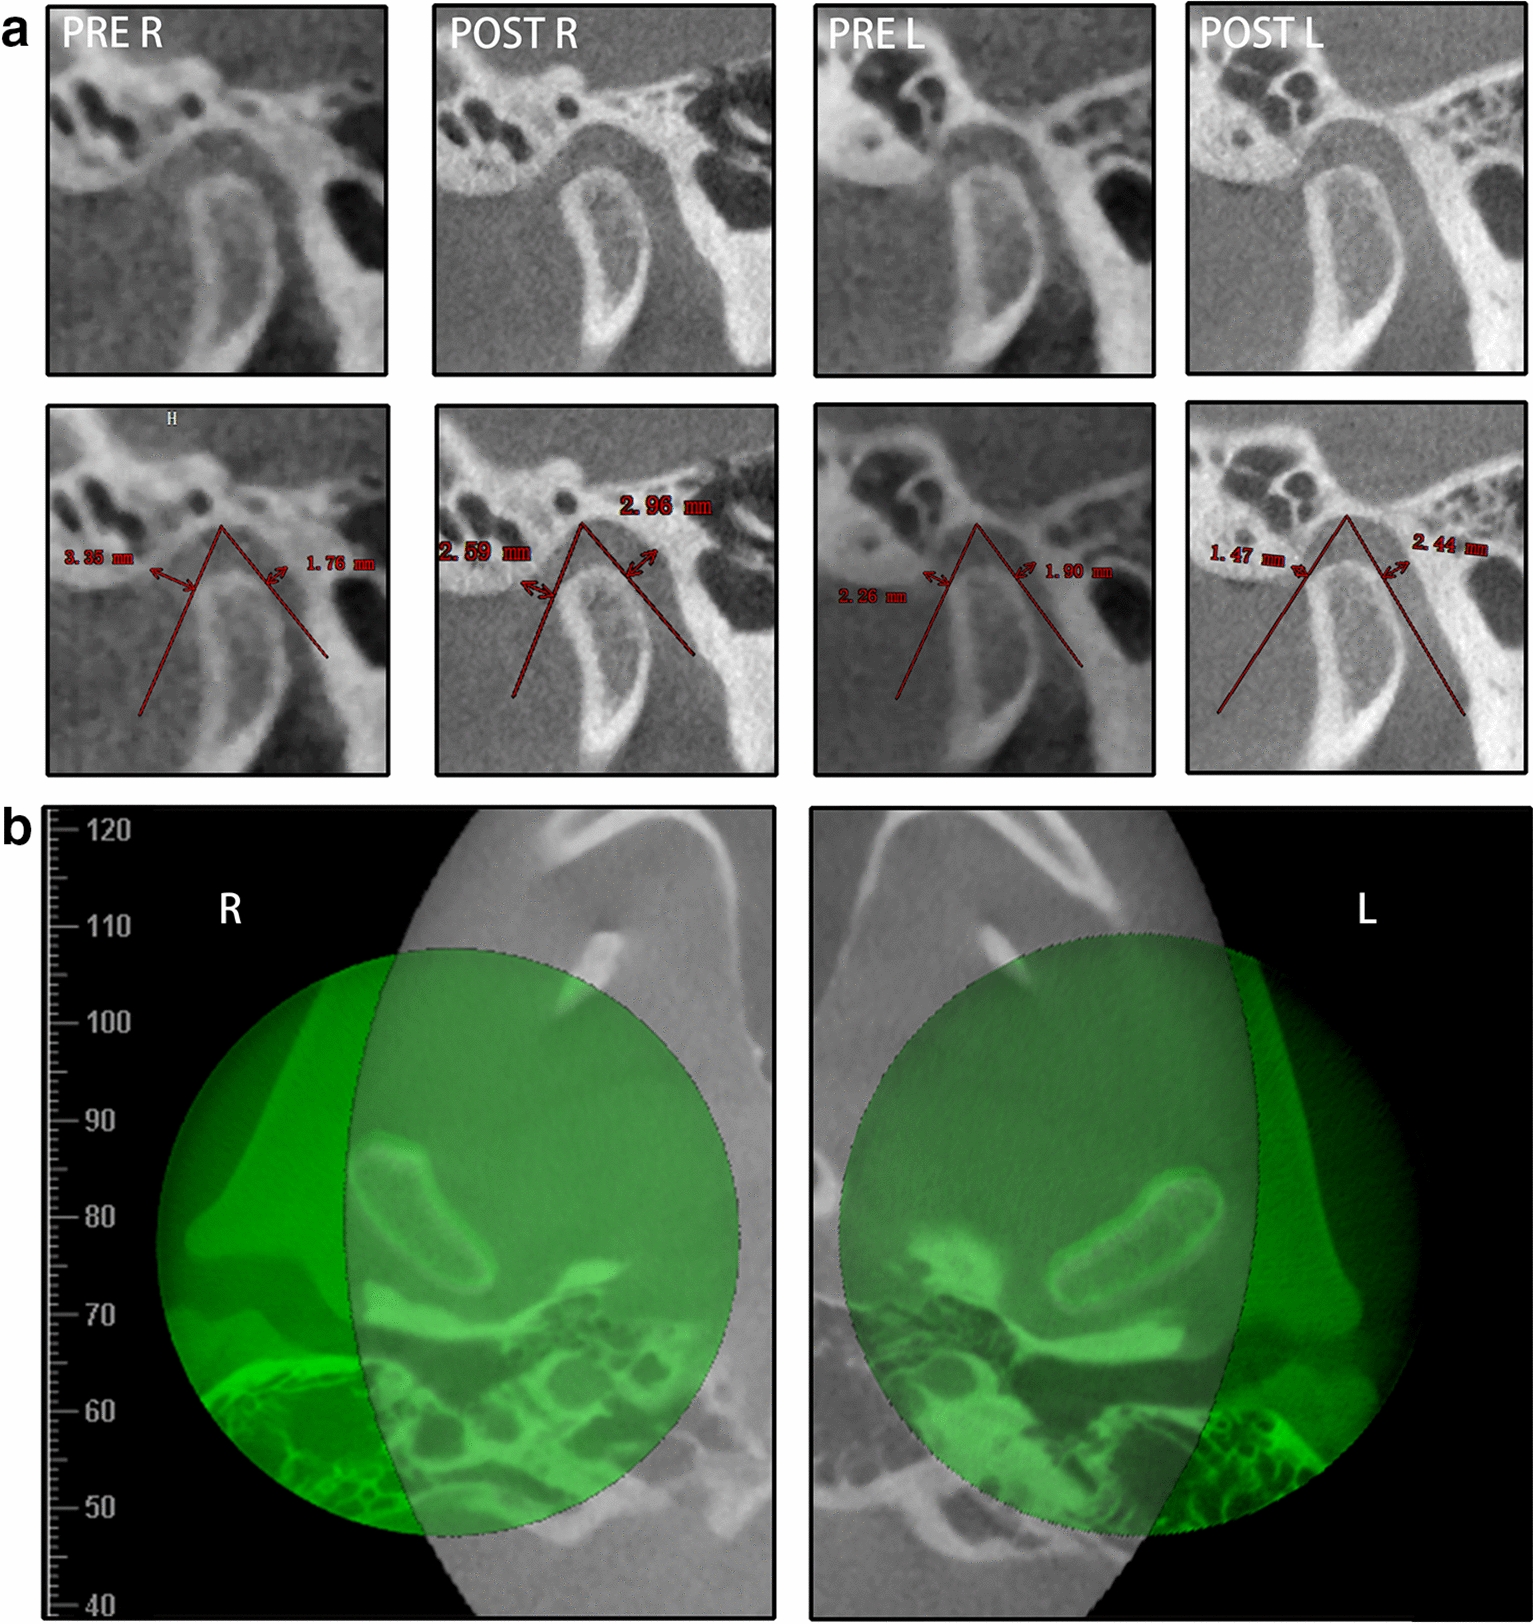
a Comparison of the pretreatment and post-treatment bilateral TMJ JSIs. b CBCT superimposition of the pretreatment (gray) and post-treatment (green) bilateral TMJs
